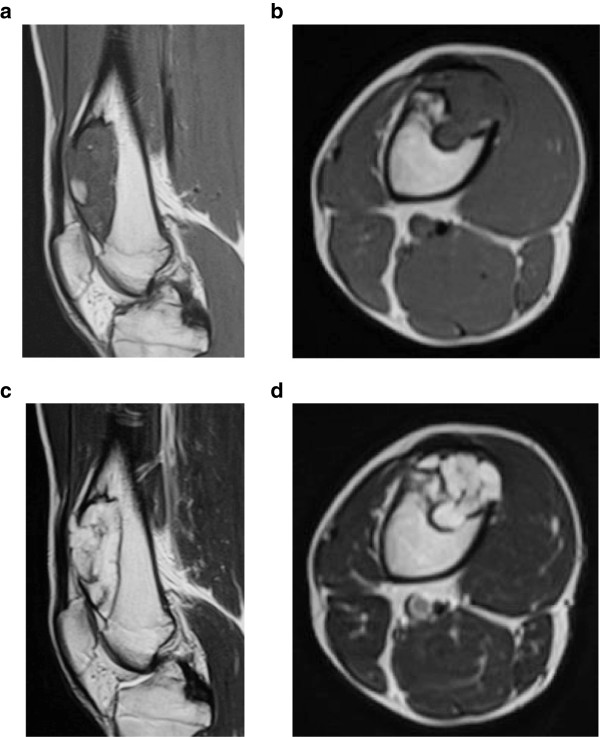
Preoperative MRI. (a) Sagittal and (b) axial T1-weighted images, showing a low-intensity mass on the anterior surface of the right distal femur. (c) Sagittal and (d) axial T2-weighted images, showing a high-intensity mass. No evidence of marrow invasion was seen.
